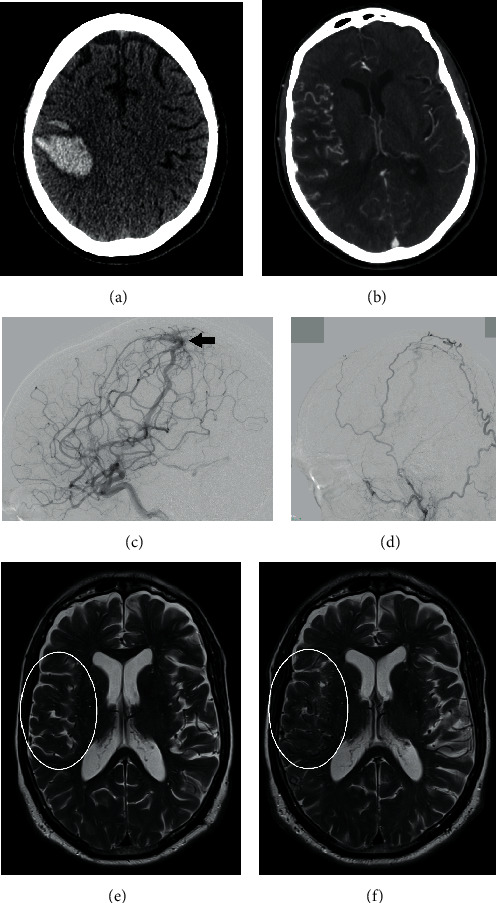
During readmission five months after the initial referral, consecutive CT scans showed multiple right-sided cerebral haemorrhages, the largest being located precentrally in the frontal lobe ((a), para-axial nonenhanced). CT angiography examination ((b), para-axial MPR) showed multiple tortuous, engorged pial veins of the right hemisphere. Cerebral angiography confirmed the presence of a suspected superficial dural arteriovenous fistula ((c), lateral projection of right internal carotid artery injection, large black arrow) in the right parietal region. The arterial feeders were mainly meningeal branches originating from the right ophthalmic artery and transosseous feeders from the right superficial temporal and occipital arteries ((d), lateral projection right external carotid artery injection); in addition, there was limited arterial supply to the fistula from the left middle meningeal artery, across the midline (not shown). Venous drainage was through a large, right temporal draining vein (large white arrow), with reflux into multiple tortuous, superficial frontotemporal veins, as shown in Figure 2(b), indicating a fistula with a high risk of haemorrhage. Comparison of axial T2-weighted images of MRI examinations at initial admittance (e) and at the second admission (f) showed the new finding of dilated pial veins (encircled), demonstrating the de novo development of the dural AV fistula.
